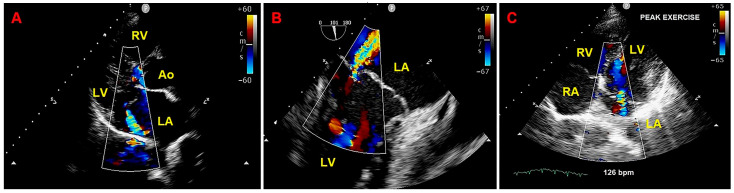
Moderate mitral regurgitation detected on resting transthoracic echocardiography from the parasternal long-axis view (A), on transesophageal echocardiography from the bicommissural view (B), and on exercise stress echocardiography from the apical four-chamber view recorded at peak exercise (C). Ao, aorta; LA; left atrium; LV left ventricle; RA, right atrium; RV, right ventricle.
